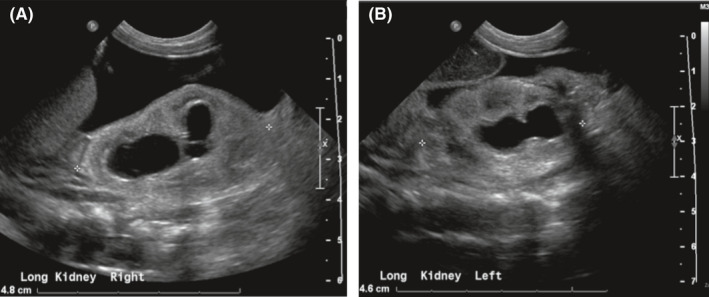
(A) Right hydronephrosis demonstrated on renal ultrasound. (B) Left hydronephrosis demonstrated on renal ultrasound
